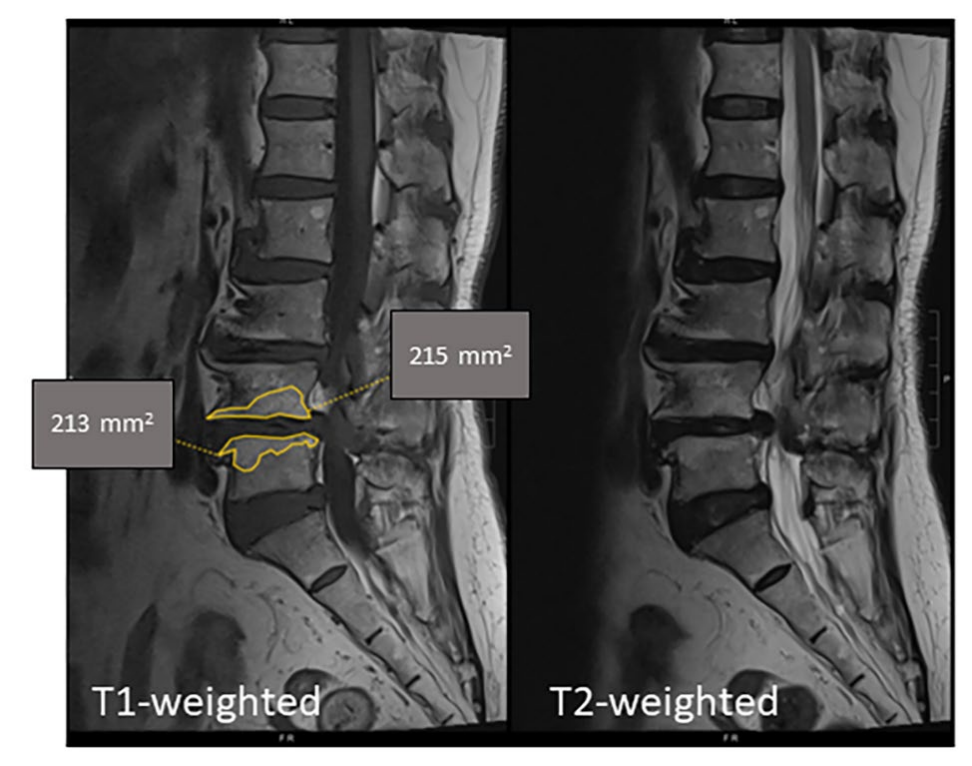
Measurement of Modic changes. T1 and T2-weighted lumbosacral spine MRI sequences in a patient with Type 2 Modic changes over the L4 and L5 vertebra. The area with Type 2 changes is demarcated in yellow. Grading of the affected area in L4 corresponds to ‘C’ since > 50% of the vertebral height is affected. Volume of MC was calculated over the worst affected vertebral level by multiplying the area of MC by the slice thickness of 4 mm
